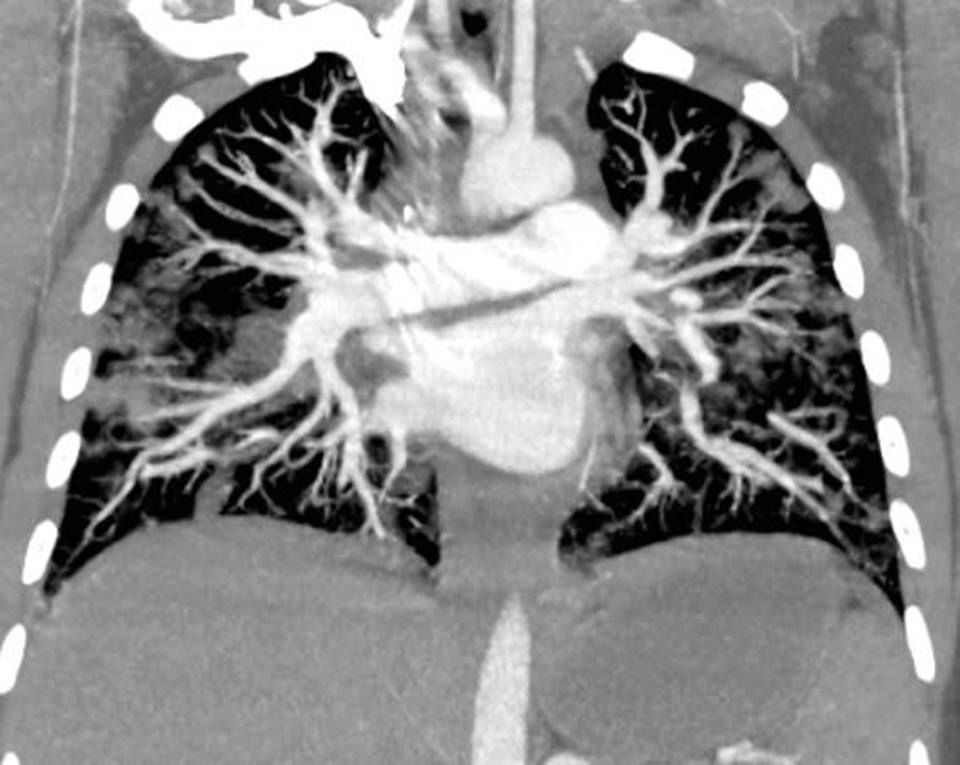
Computed tomography showing pulmonary opacities.
